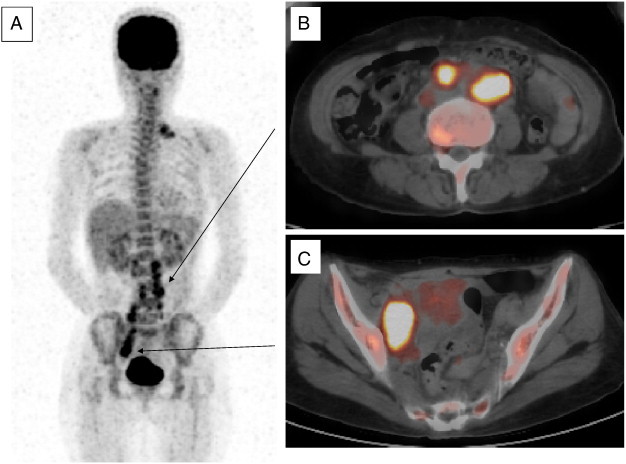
A coronal 18F-fluorodeoxyglucose (FDG)-positron emission tomography scan showing significant FDG uptake in the (A) retroperitoneal and supraclavicular lymph nodes, (B) paraaortic lymph nodes, and (C) pelvic lymph node.
